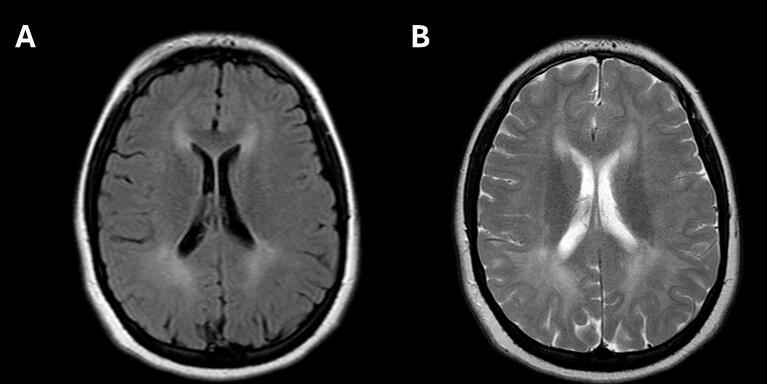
White matter abnormalities on magnetic resonance imaging: FLAIR sequence (A) and T2-weighted sequence (B).
